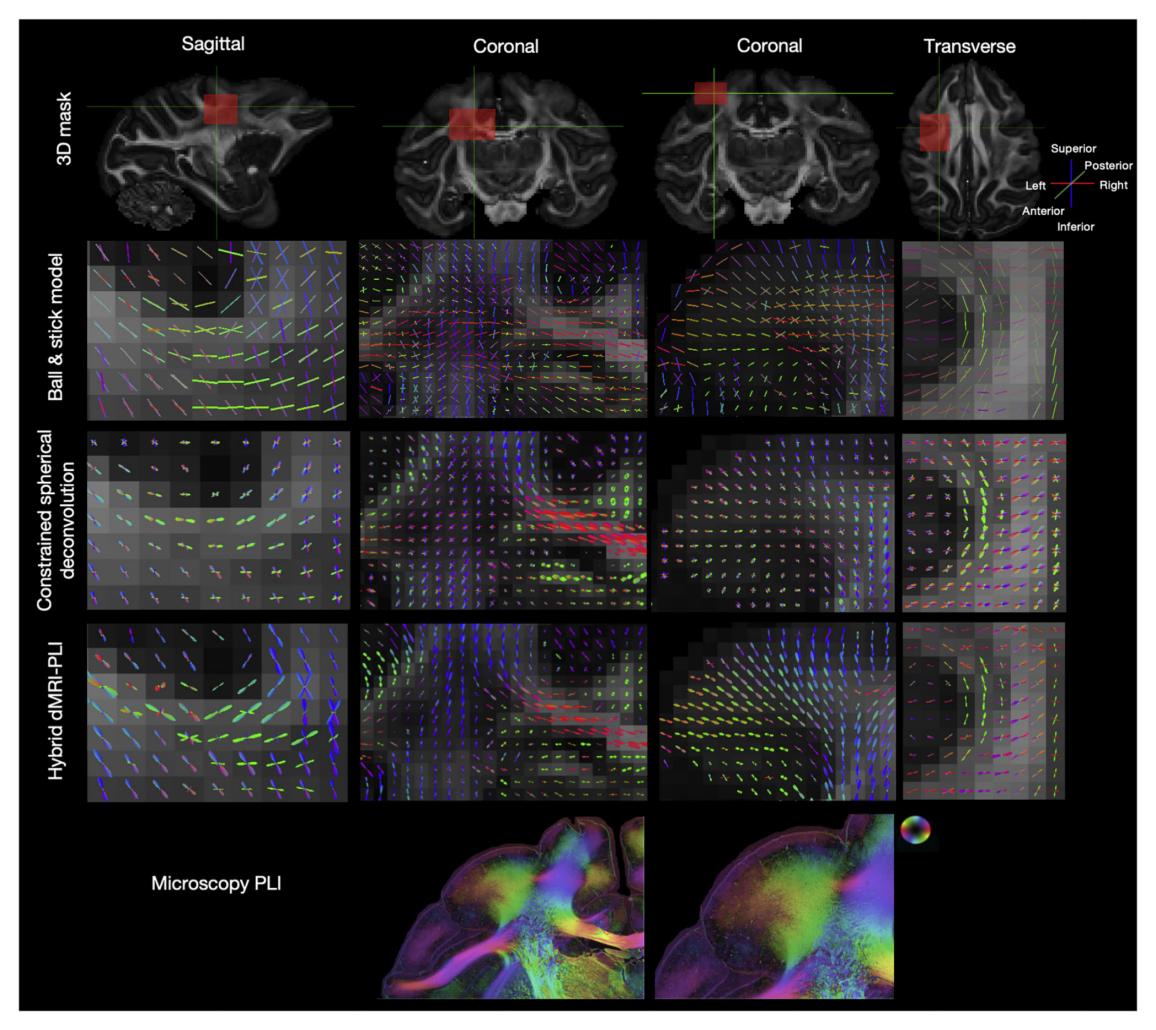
Comparison between dMRI FOD and hybrid MRI-PLI FOD. The FOD generated from the Ball and Stick model (Top), constrained spherical deconvolution (Middle) and hybrid MRI-PLI (Bottom). The orientations in sagittal, coronal and transverse views are presented for U-fibres (left and right), a region covering the corpus callosum and centrum semiovale (middle left) and cortex (middle right). The PLI hue-saturation-value with the colour-coded orientations is provided at the bottom, and the contrast has been edited to highlight the grey matter. Note the colour schemes for the FODs and PLI are not equivalent.
